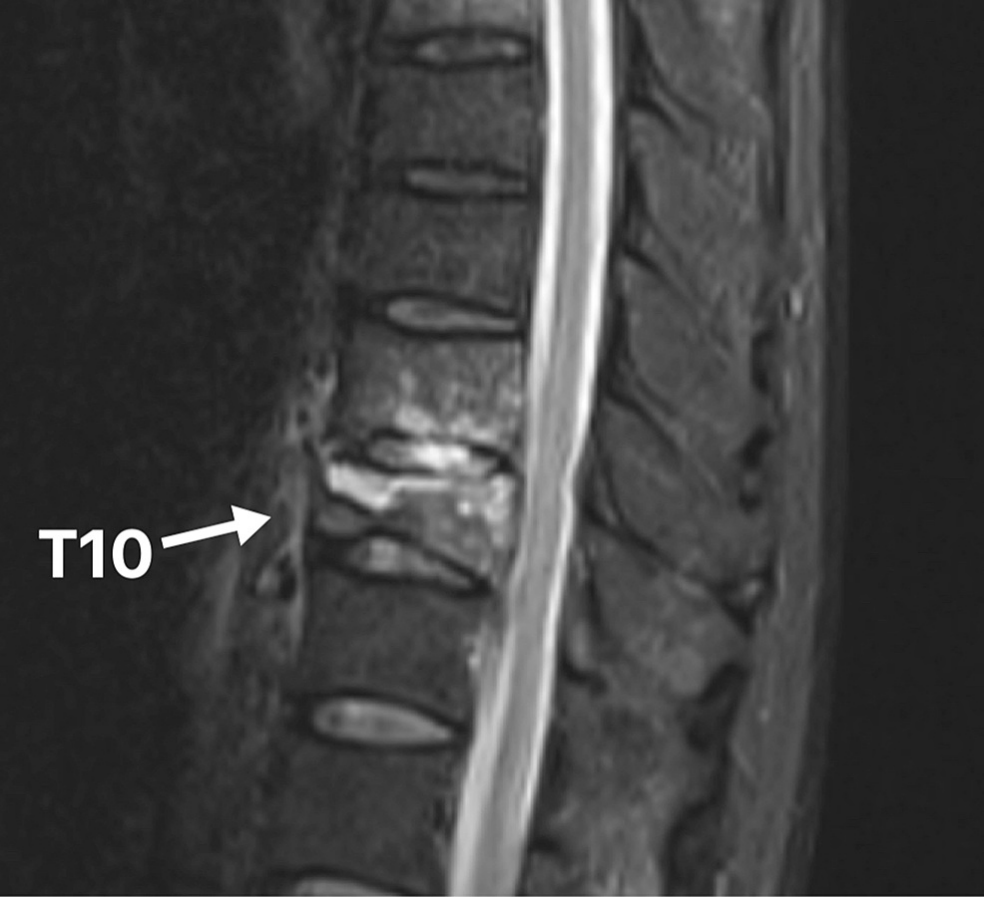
MRI spine sagittal view revealed hyperintense lesion over T9 and T10 vertebral bodies.
